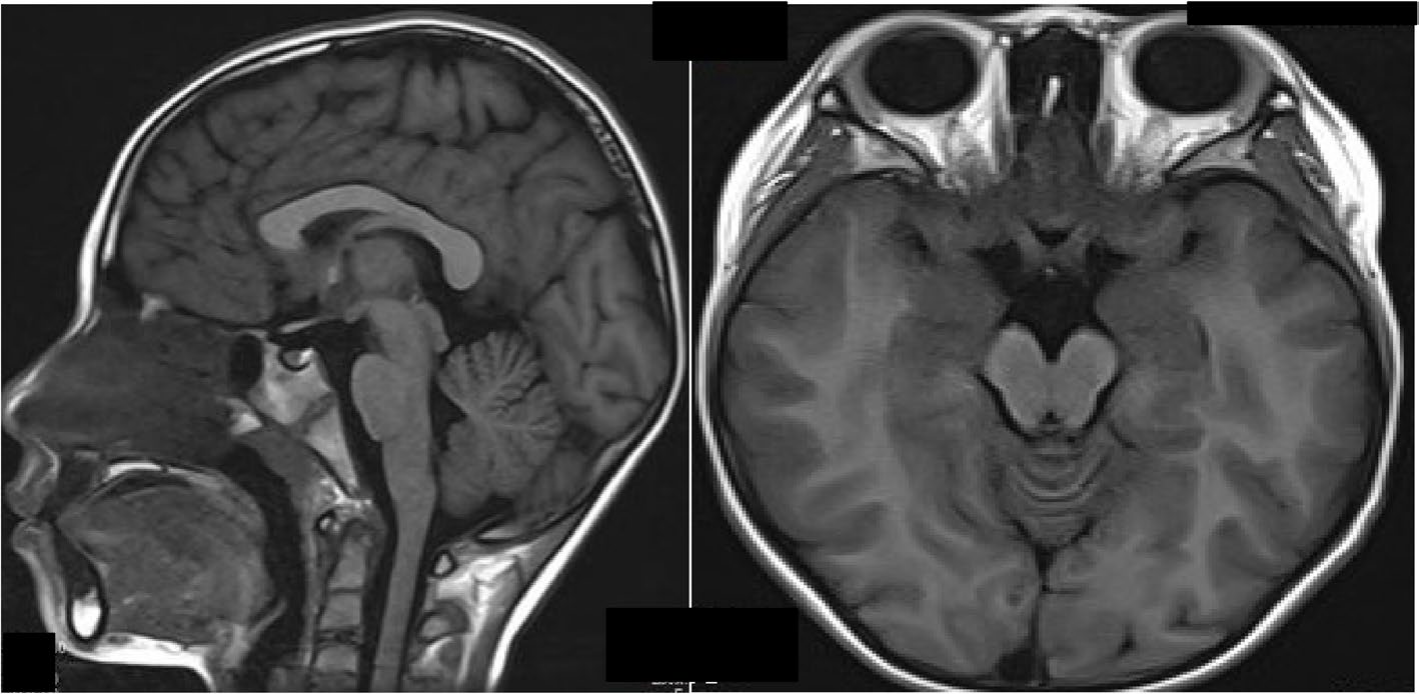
MRI scans of the patients as obtained from the hospital
